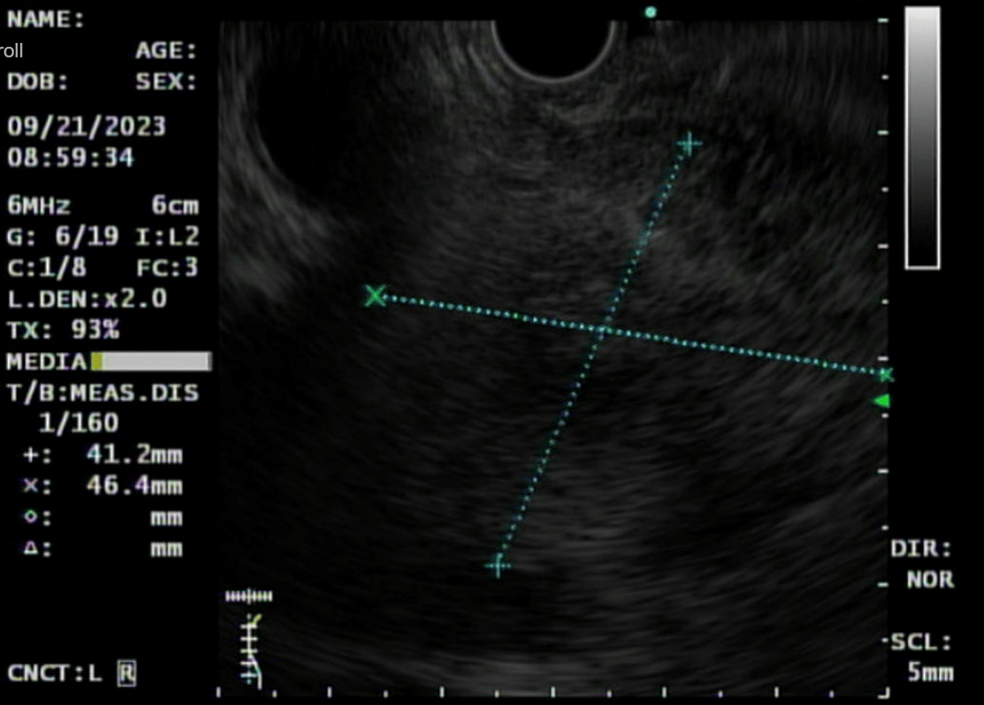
Endoscopic ultrasound findings of a 4.5-centimeter fullness in the head of the pancreas are isoechoic to the remainder of the pancreas.
